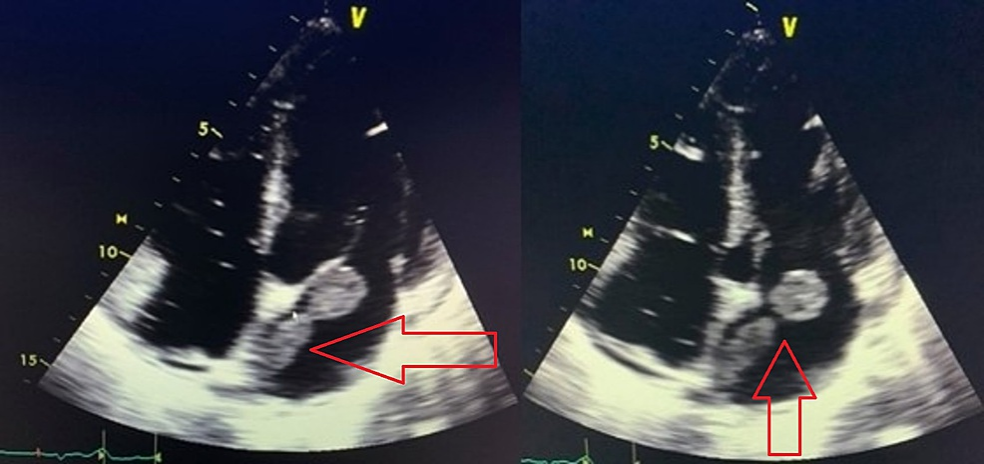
Transesophageal echocardiogram showing a large and elongated mass of 6 x 2 cm that stretched from the right upper pulmonary vein into the left atrium, suggestive of heart invasion.
